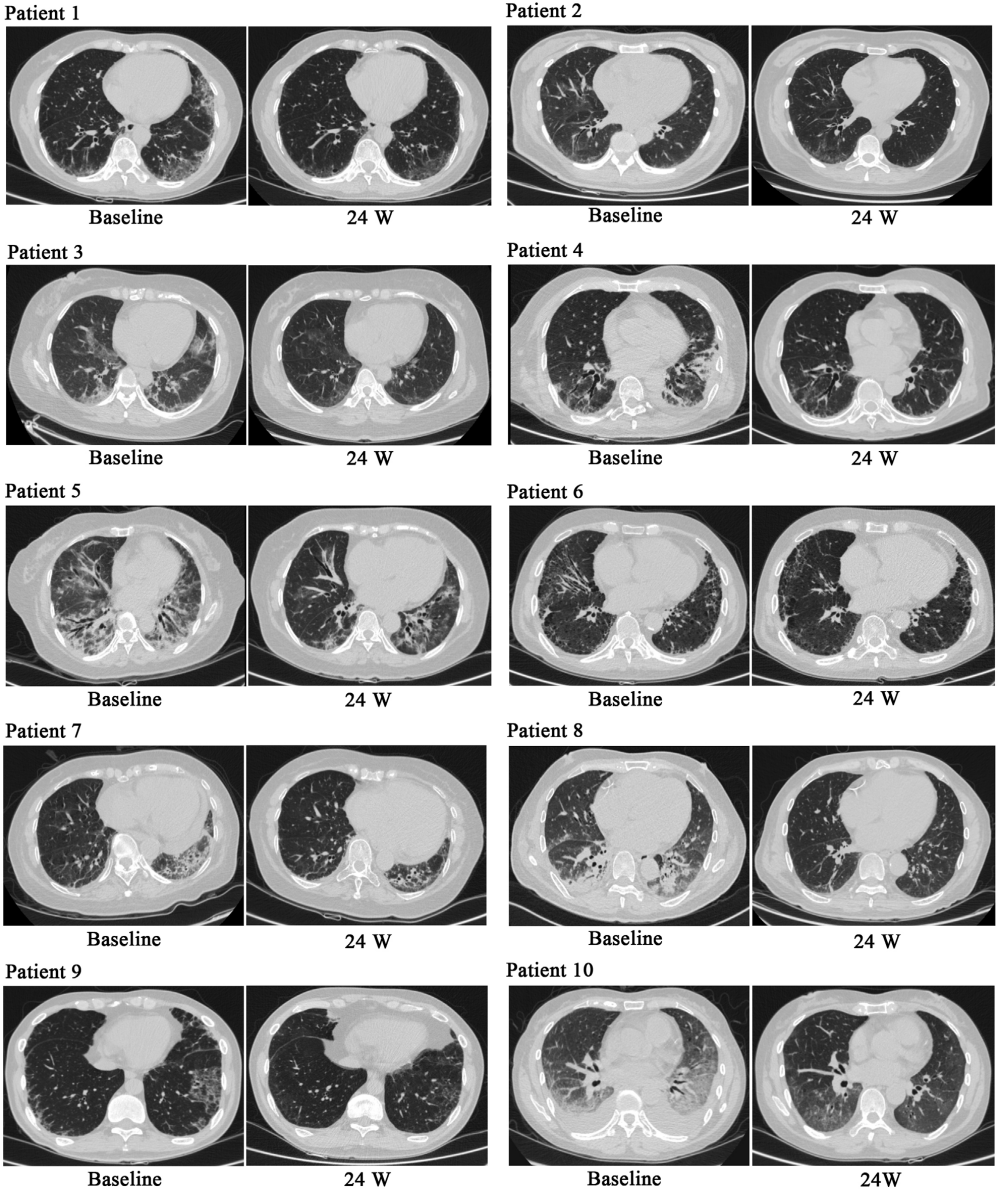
Chest HRCT before and after treatment. After treatment, chest HRCT scans demonstrated significant improvements compared with baseline.
